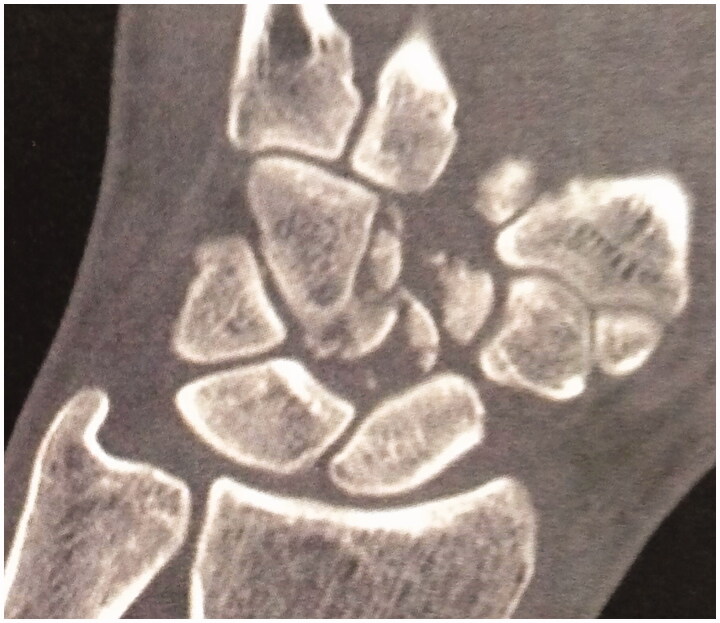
Pre-operative CT-scan case 2.
